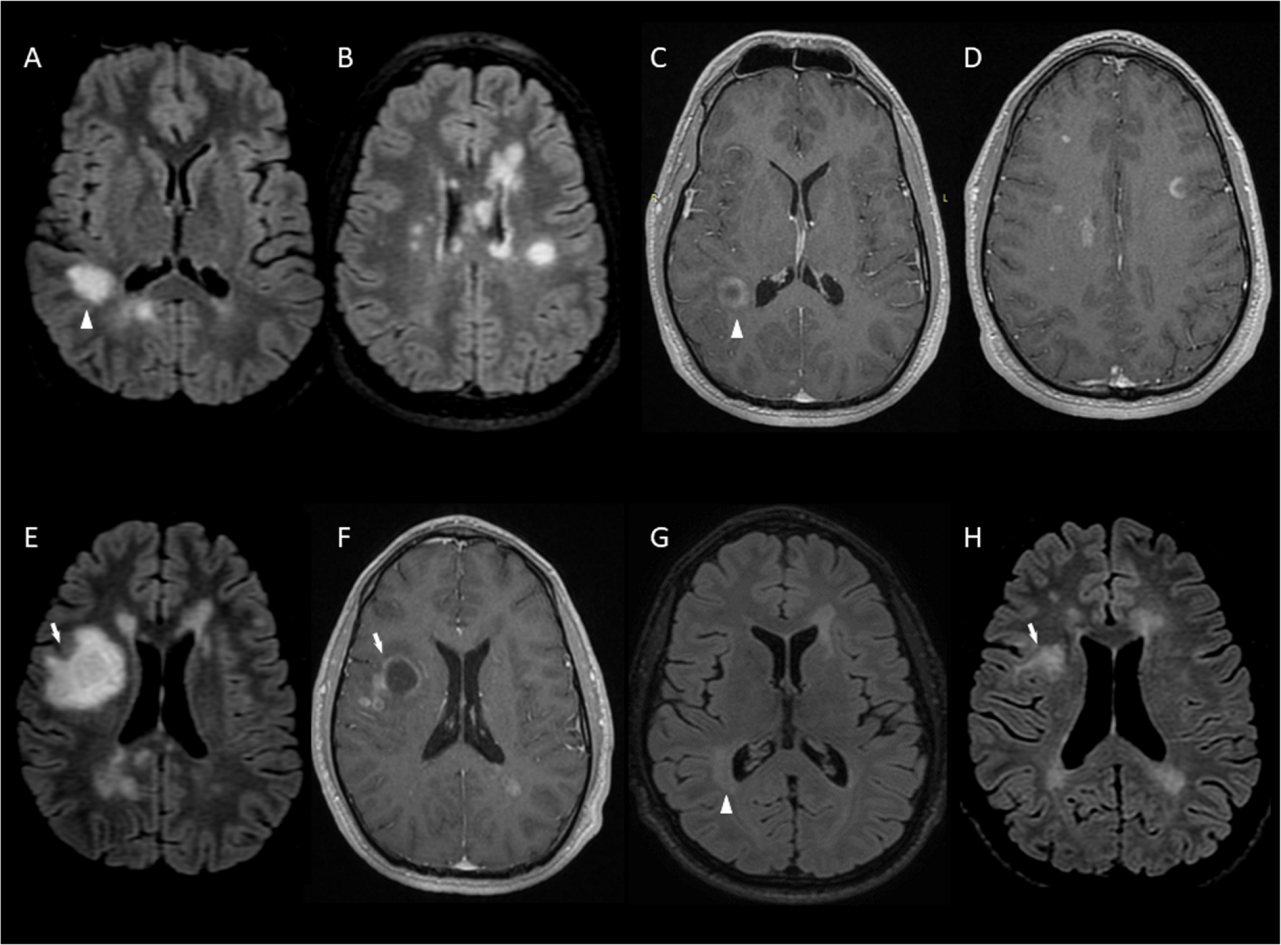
MRI of Case 6 showing coexistence of tumefactive and BCS-type lesions. At symptom onset a tumefactive lesion with incomplete ring Gd + in the right optic radiation-parietal lobe (A and C arrowhead) and multiple lesions (B); 14 with Gd + (D). A BCS-type lesion with incomplete ring Gd + (E and F thick arrow) in the right corona radiata evolved at a 2-months follow-up. BCS-type (H thick arrow) and tumefactive (G arrowhead) lesions at month 6 after rituximab initiation, one year from the first clinical episode. a, b, e, g, h: 3D FLAIR images. c, d, f: 3D T1-weighted contrast-enhanced images. BCS: Baló’s concentric sclerosis, Gd+: gadolinium enhancement
